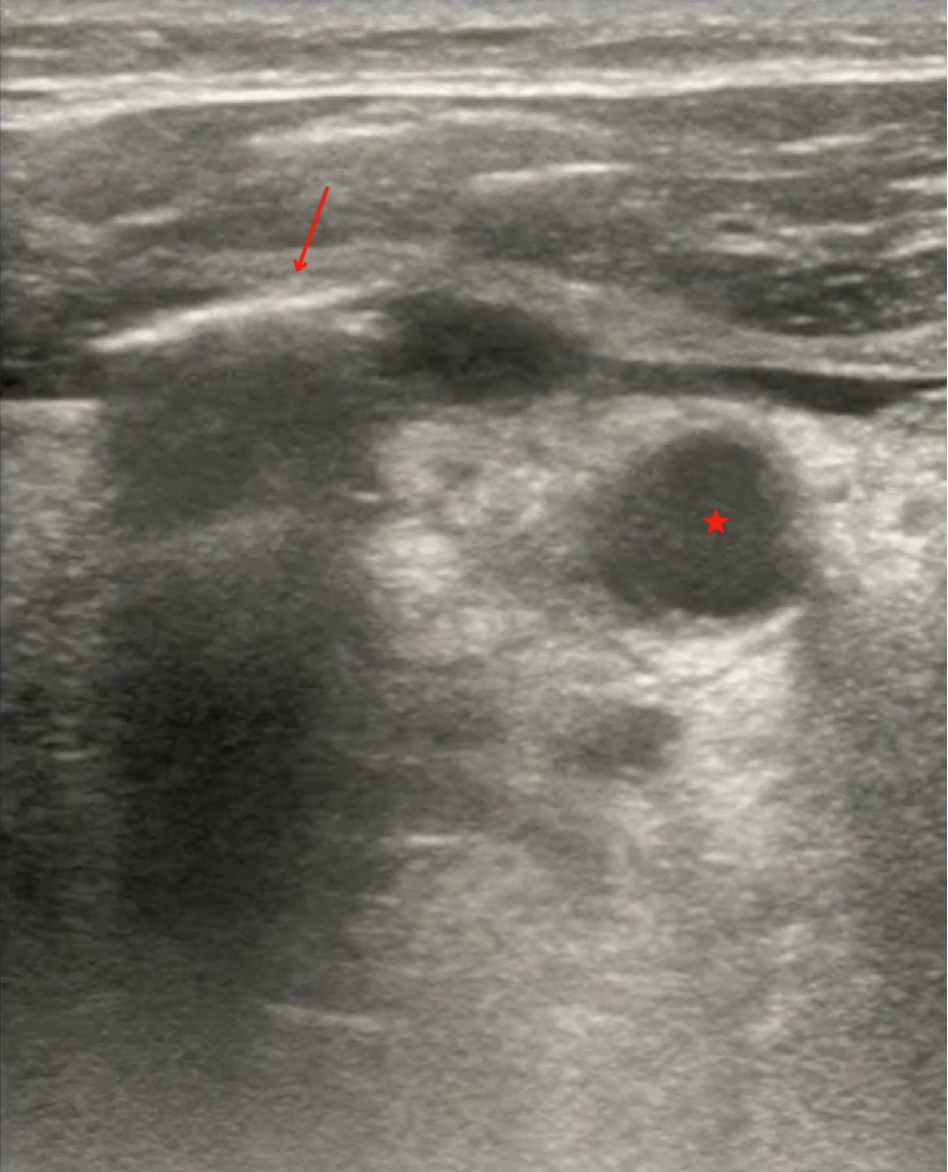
Bedside ultrasound showed shadowing under an area of subcutaneous air (arrow) adjacent to the trachea (star).
